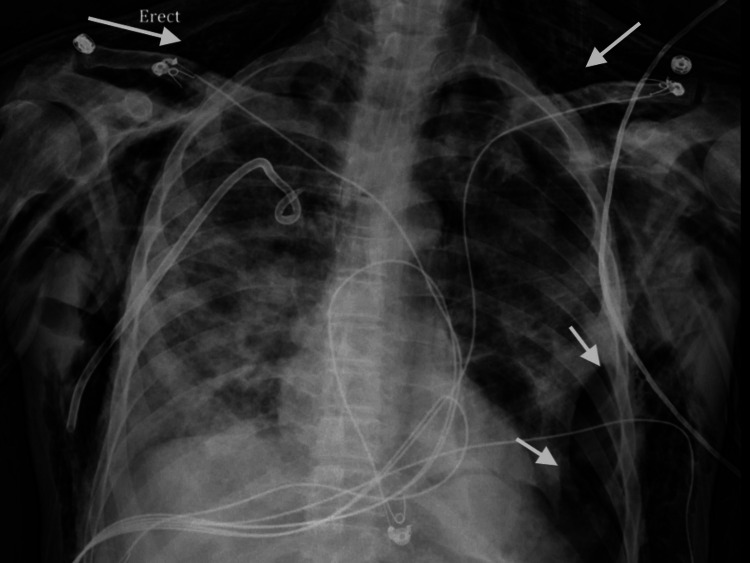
Repeat CXR during the hospital course The image shows small-to-moderate-sized left basilar pneumothorax and diffuse subcutaneous emphysema (arrows) CXR: chest X-ray
